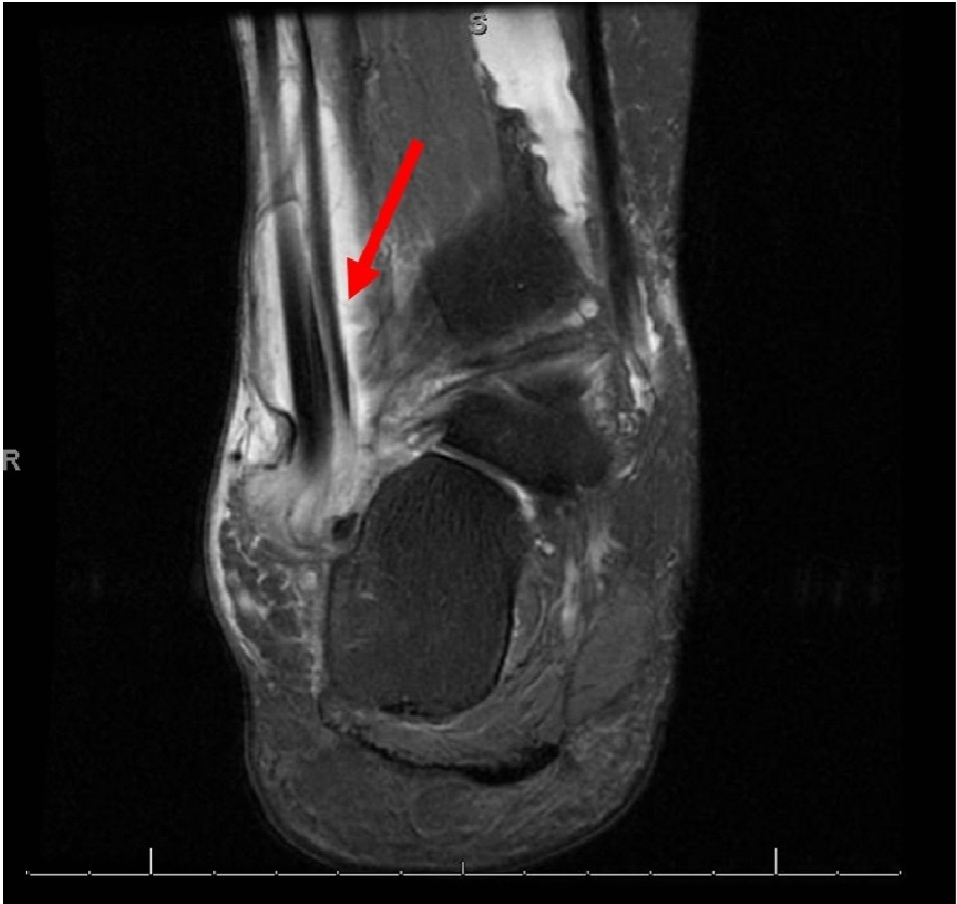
Coronal 3T MRI of the right foot. Severe peroneal tenosynovitis with interstitial peroneal tendon tearing (arrow). MRI: Magnetic resonance imaging.
